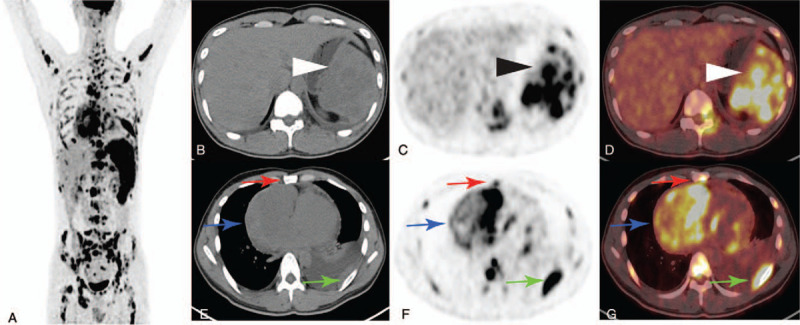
The maximum intensity projection of ^18^F-FDG PET imaging (A) revealed diffused intense hypermetabolic masses in the whole body. Transaxial PET/CT images manifested splenomegaly, filled with intense hypermetabolic nodules and masses (B–D, arrowhead). Multiple regions of increased ^18^F-FDG uptake were observed in the mediastinum (E–G, blue arrows), left pleura (green arrows), and bones (red arrows). FDG = fluorodeoxyglucose, PET/CT = positron emission tomography/computed tomography.
